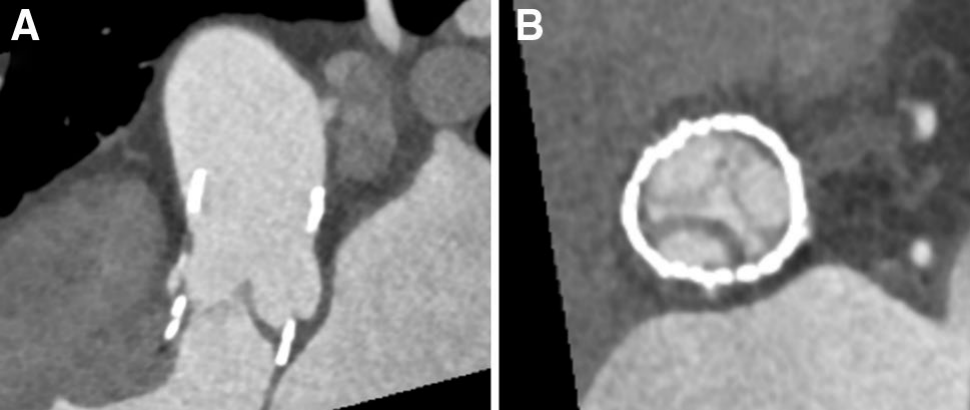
Cardiac computed tomography multiplanar reformatted reconstructions of a perceval sutureless aortic valve bioprosthesis in mid-diastole. One cusp was markedly thickened with hypo-attenuated leaflet thickening (panel A). The three valve leaflets are shown simultaneously: two of them normal and one cusp with hypo-attenuated leaflet thickening (panel B)
